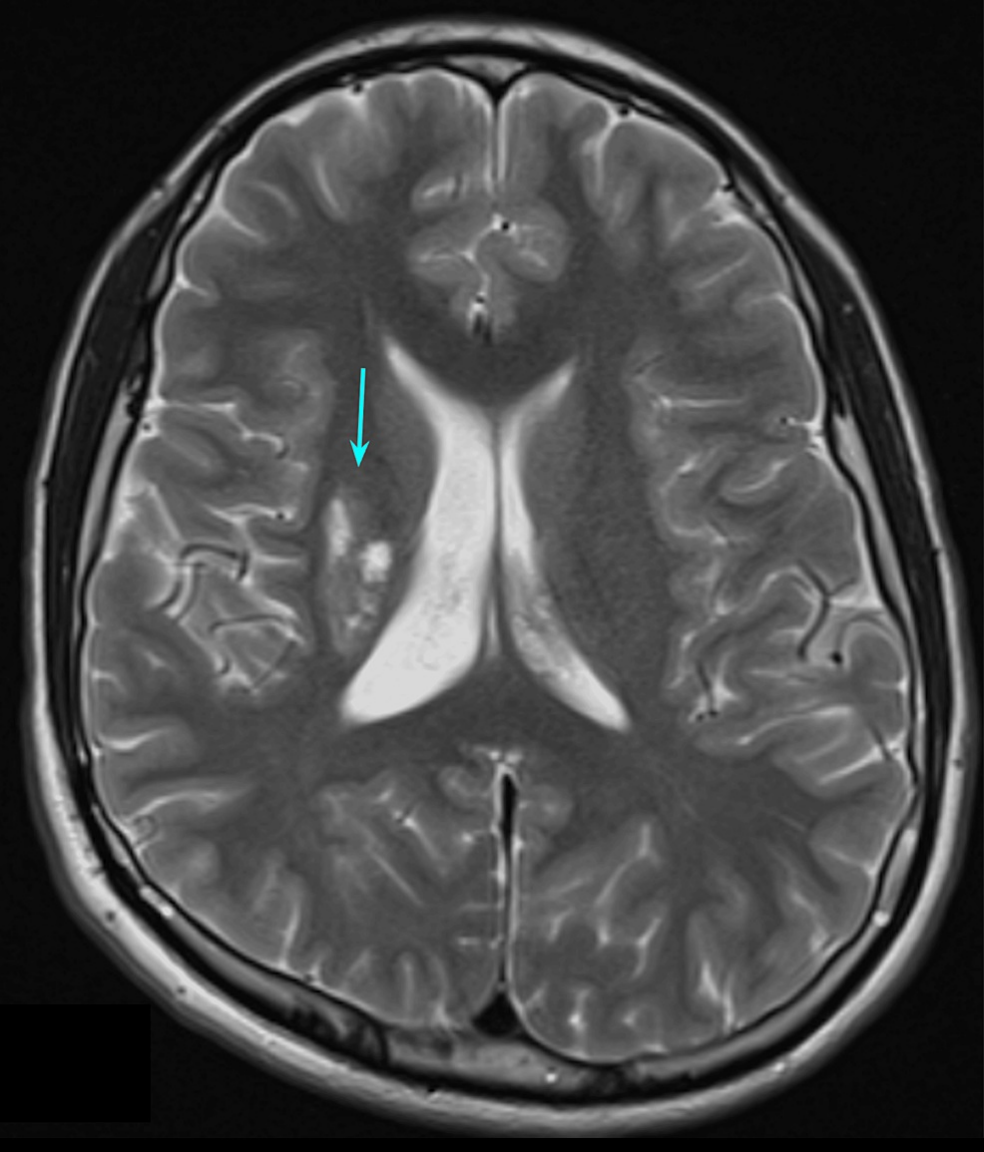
Second brain MRI T2 image Chronic changes associated with the ischemic process, with major involvement of the lentiform nucleus (blue arrow).
